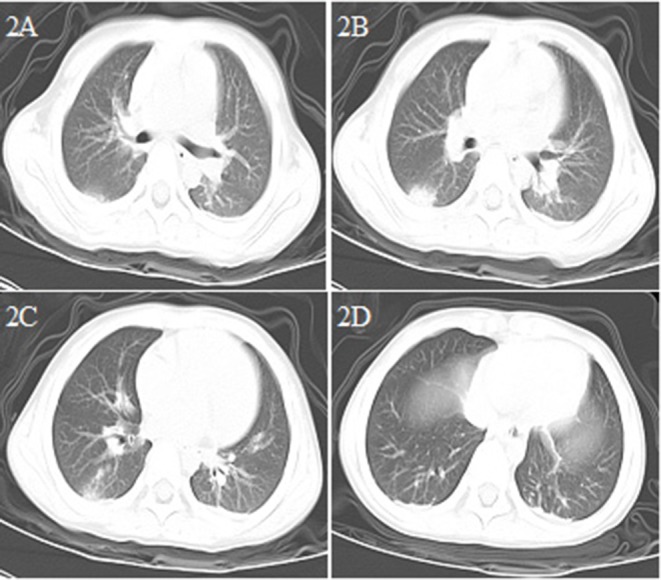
Chest CT images for case 2 showing a round-like mass shadow in the dorsal segment of the lower right lobe with a slight ground-glass opacity on the edge, a strip shadow in the local area of the left lower lobe, and an arc dense under the bilateral pleura on day 3 after symptom onset (A–D).
